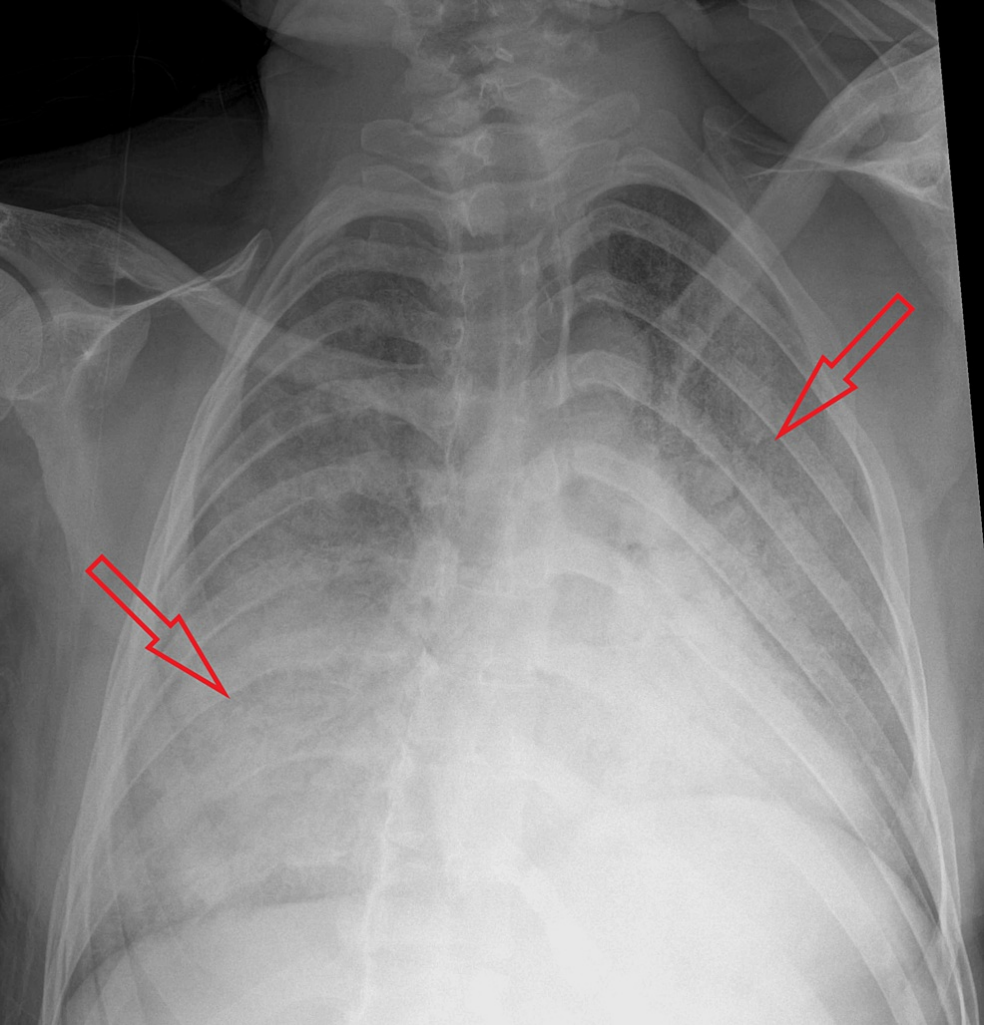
Chest X-ray after the seizure episode showing bilateral infiltrates.
